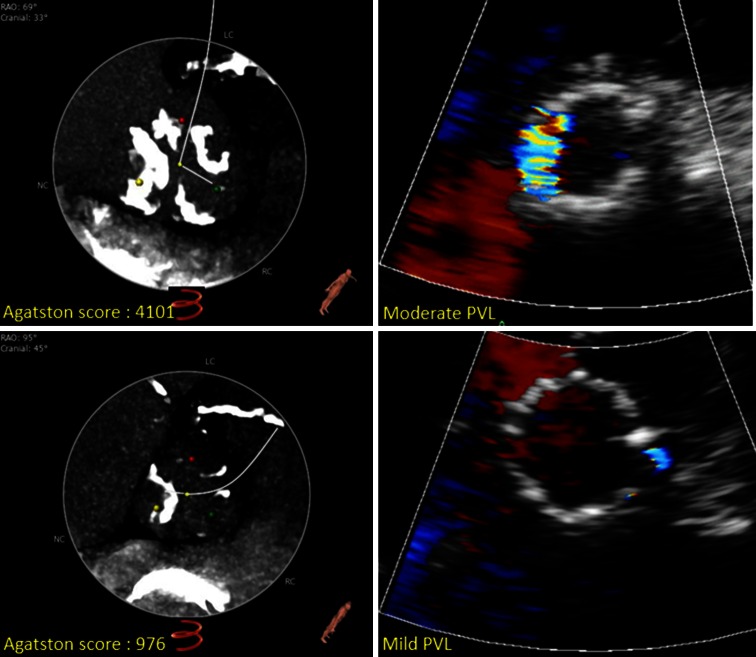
Examples from two patients with low and high Agatston score, respectively and corresponding *colour* Doppler short-axis views of paravalvular leak on 2D echocardiography
